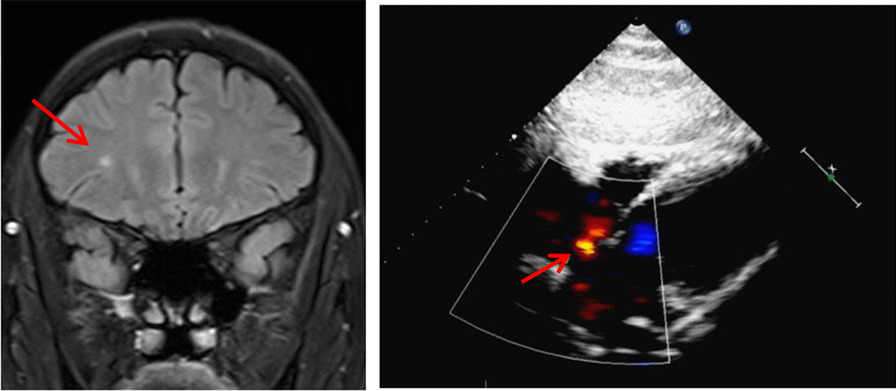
Example of a case with PFO and one brain ischemic lesion. One Tibetan male patient with age 32 years, is a resident in an area over 3200 m above sea level with a long-term headache symptom. Left panel, brain MRI shows high-intensity signal by a coronal section on FLAIR. Right panel: echocardiography imaging of the heart. Arrow points to PFO with a diameter of 3.4 mm
